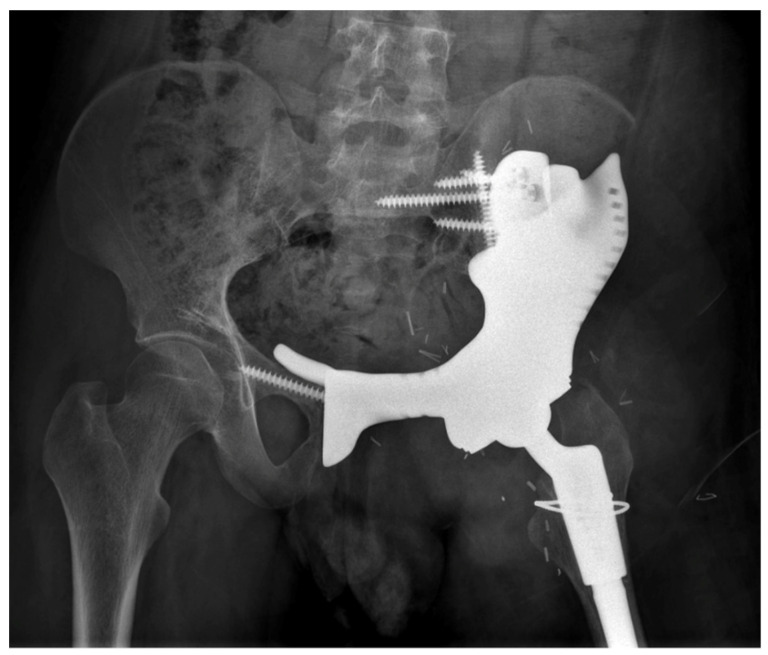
X-ray of a custom prosthetic reconstruction, for a type 2 resection of a grade 2 chondrosarcoma.
